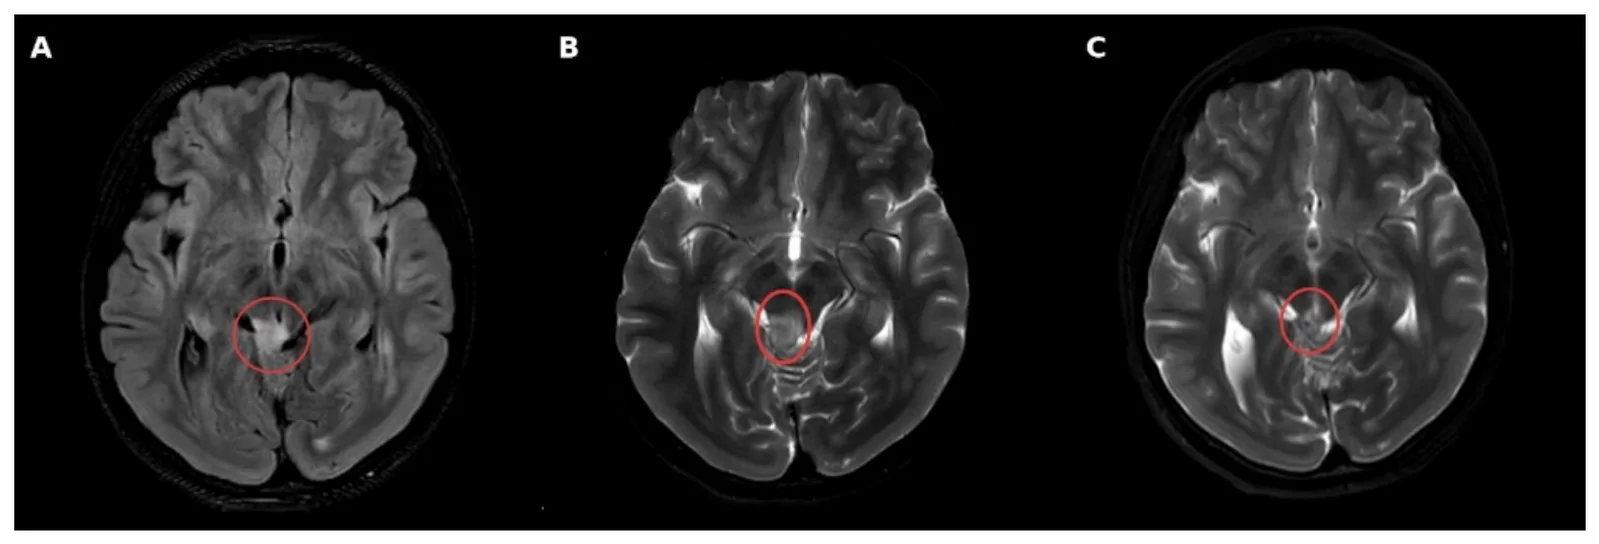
Brain Magnetic Resonance Imaging (MRI) features of the low-grade tectal glioneuronal tumor. (A) Axial Fluid-Attenuated Inversion Recovery (FLAIR) image demonstrates a well-circumscribed, hyperintense expansile lesion located in the tectal plate, with adjacent periventricular white matter signal change (red circle) (Pre-op). (B) Axial T2-weighted image shows an intrinsically hyperintense tectal mass (red circle) protruding into the quadrigeminal cistern, with narrowing of the cerebral aqueduct and no significant surrounding perilesional vasogenic edema (Pre-op). (C) Postoperative axial T2-weighted follow-up image depicts significant surgical debulking with a small, stable focus of residual tumor/postoperative changes along the tectal margin (red circle), and decompression of adjacent CSF pathways without significant surrounding edema.
